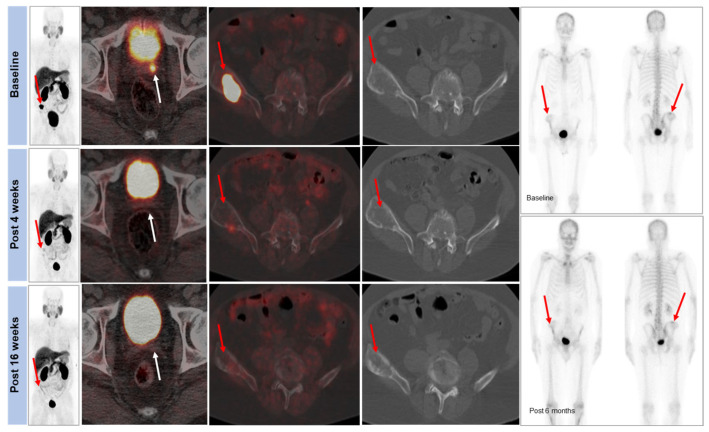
Response heterogeneity—changes in activity of lesions within individual patients at different times—detected by using [^18^F]D4-FCH PET/CT. Representative PET images acquired at baseline (t1), early post-treatment (4–6 weeks; t2), and midtherapy (14–16 weeks; t3) demonstrating decreasing radiotracer uptake in a left seminal vesicle lesion (white arrows) and right iliac bone metastasis (red arrows).
